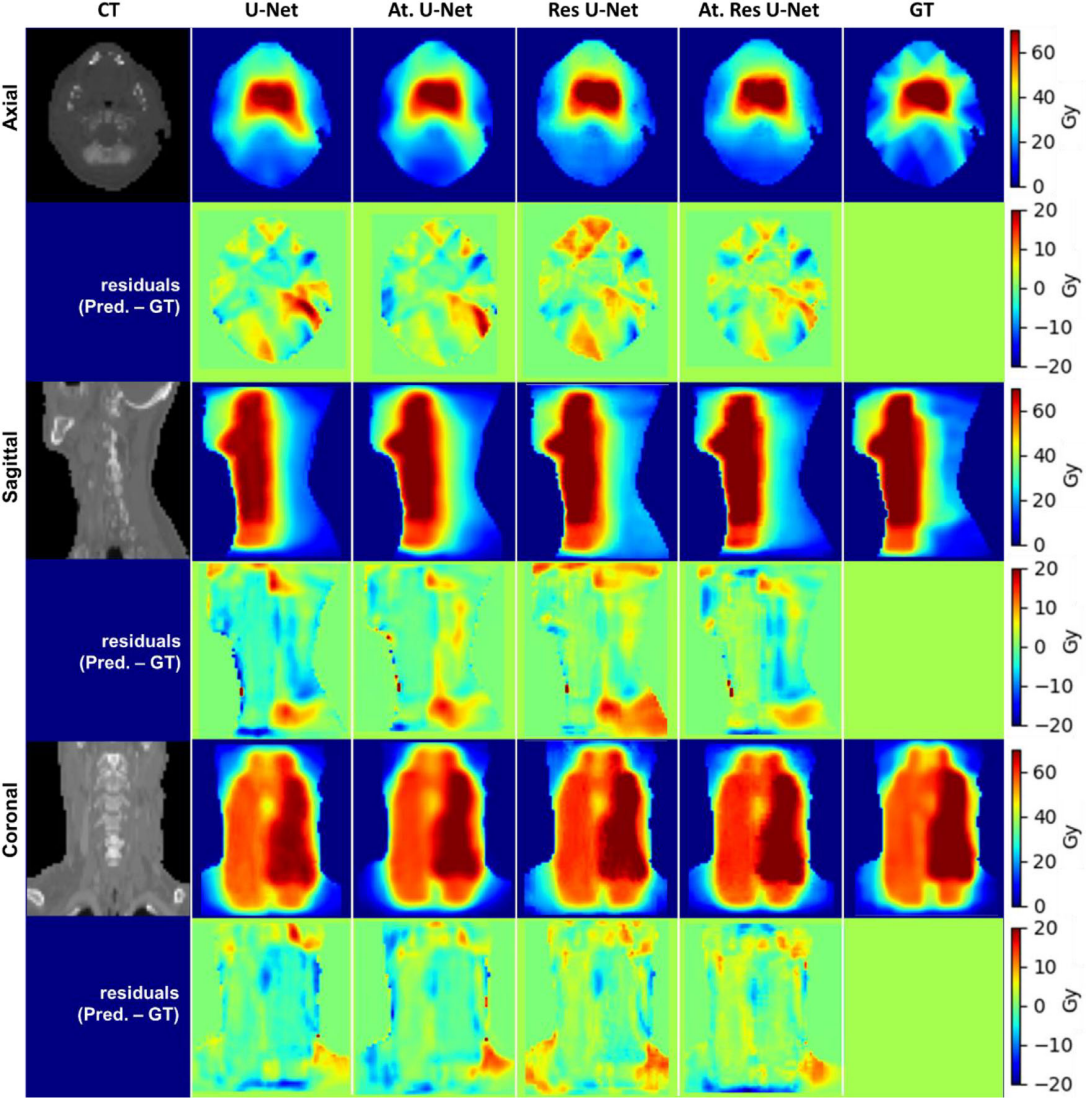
An example of head and neck KBP dose distribution prediction results side‐by‐side with the ground‐truth displayed on different views (axial, coronal, and sagittal) for one patient in the test set. Columns demonstrate the CT image and dose distribution results obtained by 3D U‐Net, 3D attention U‐Net, 3D Res U‐Net, 3D attention Res U‐Net, and the ground‐truth, respectively. Rows indicate different views and the corresponding dose difference maps.
